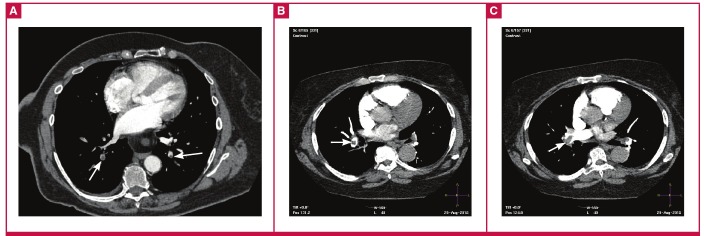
The CTPA appearances, showing the different degrees of obstruction in the lobar and segmental arteries, are depicted in the series of images. (A) Axial CT scan showing partially occlusive thrombi (short arrows) in the right and left lower lobe basal segmental arteries. (B) Axial CT scan showing partially occlusive thrombus (long arrow) in the right lower lobe artery. (C) Axial CT scan showing totally occlusive thrombus (long arrow) in the right lower lobe artery.
